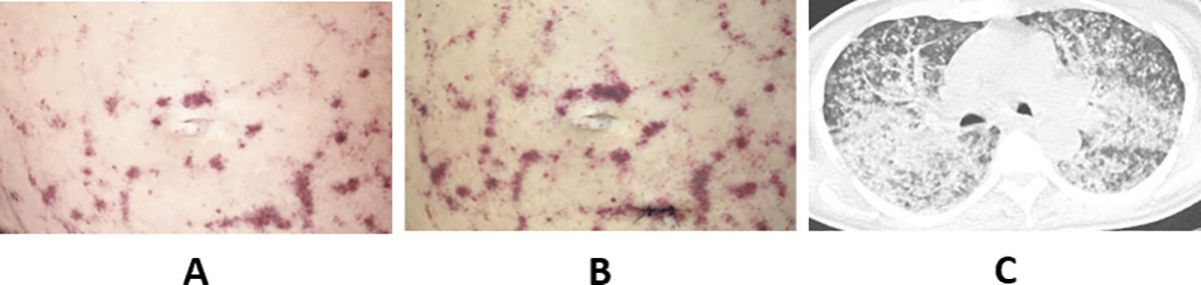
(A) Periumbilical skin lesions at Day 1. (B) Periumbilical skin lesions at Day 2. (C) Multiplanar reconstruction of thoracic CT scan showing bilateral alveolar and interstitial lung infiltrates, with multiple patterns including consolidations, nodules, ground-glass, and tree-in-bud opacities.
